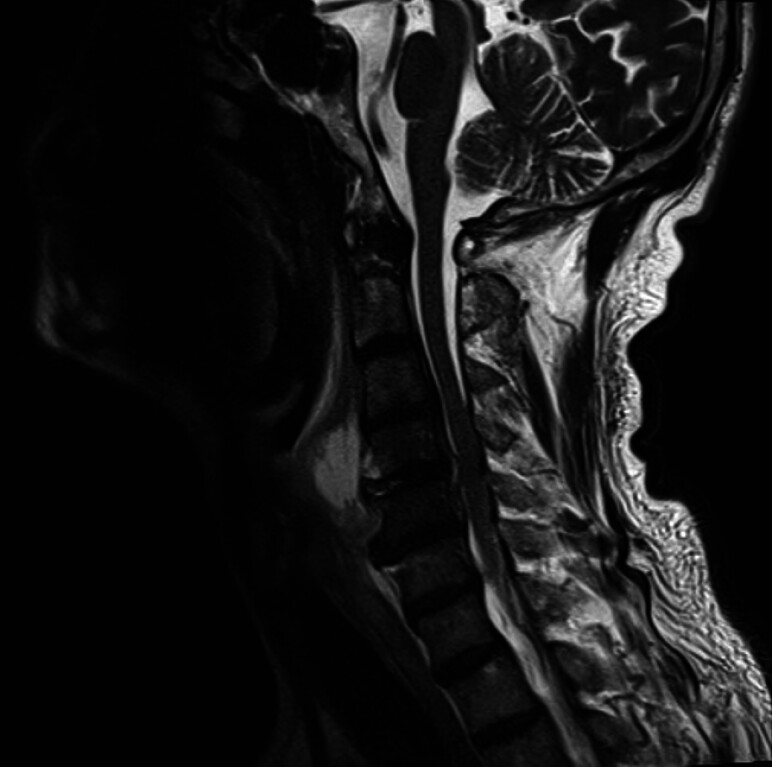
Cervical MRI revealing a prevertebral abscess at C4.
